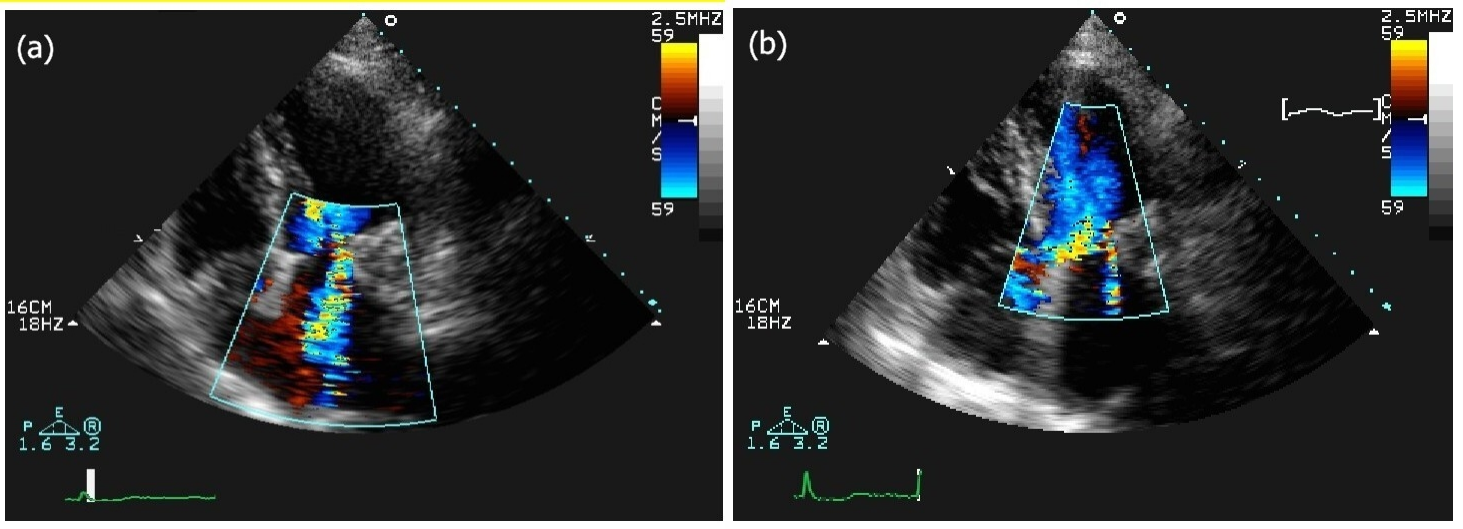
Two-dimensional echocardiogram, apical four-chamber view, colour Doppler. (a) Pre-operative: moderate to severe mitral regurgitation is present. (b) Postoperative: trivial mitral regurgitation is seen in the left atrium.
